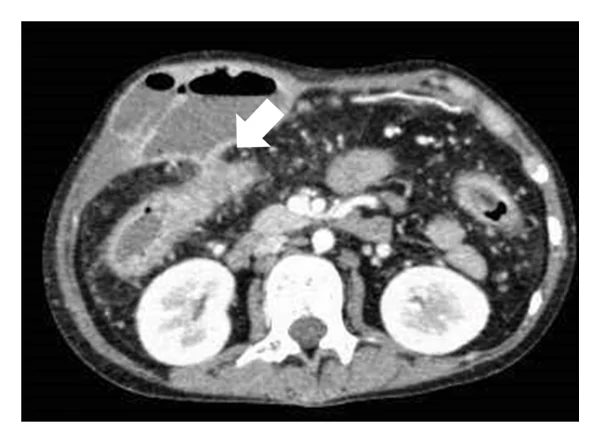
Contrast‐enhanced computed tomography demonstrating a fistulous communication between the abscess cavity and the transverse colon (arrow), with circumferential thickening of the colonic wall.
